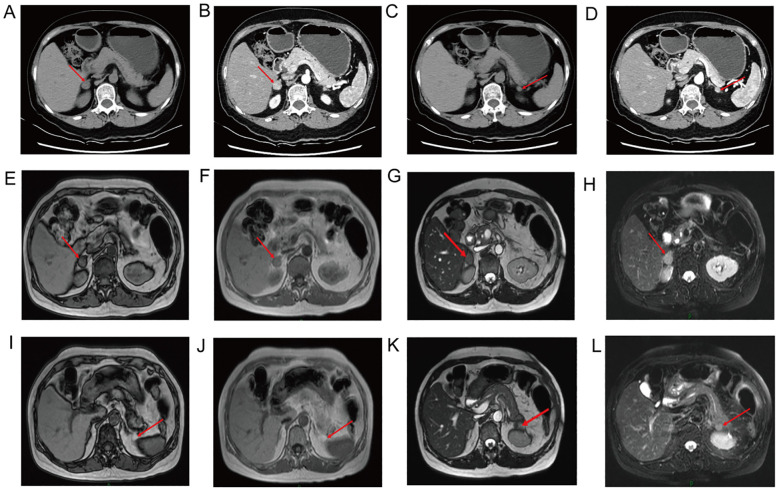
Multimodal imaging of the adrenal glands in Case 2. (A) Unenhanced CT demonstrating an19×18mm hypodense nodule in the right adrenal gland, with an attenuation value of 22 HU (arrow). (B) Contrast-enhanced CT demonstrating an19×18mm hypodense nodule in the right adrenal gland. (C) Unenhanced CT demonstrating an 10x11 mm hypodense nodule in the left adrenal gland, with an attenuation value of 9 HU (arrow). (D) Contrast-enhanced CT demonstrating an 10x11 mm hypodense nodule in the left adrenal gland (arrow). (E) T1-weighted out-of-phase MRI of the right adrenal gland. (F) T1-weighted in-phase MRI of the right adrenal gland. (G) T2-weighted MRI of the right adrenal gland. (H) Fat-suppressed T2-weighted MRI of the right adrenal gland. (I) T1-weighted out-of-phase MRI of the left adrenal gland. (J) T1-weighted in-phase MRI of the left adrenal gland. (K) T2-weighted MRI of the right adrenal gland. (L). Fat-suppressed T2-weighted MRI of the left adrenal gland. CT, computed tomography; MRI, magnetic resonance imaging; HU, hounsfield unit.
